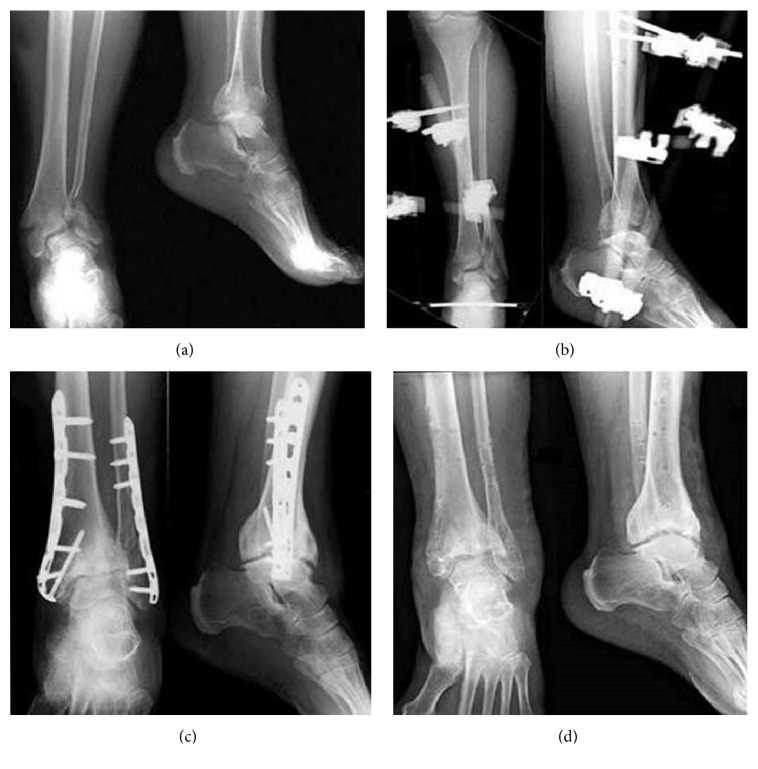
Case N*°* (47) of Table 2:* a 68-year-old man with a 43-B3 closed fracture treated with two-stage* ORIF technique:* (a) preoperative radiographic image; (b) after prefix implant, radiographic image; (c) definitive implant after 16 days, 1-month follow-up; (d) radiographic aspect at 37-month follow-up after implant removal.
